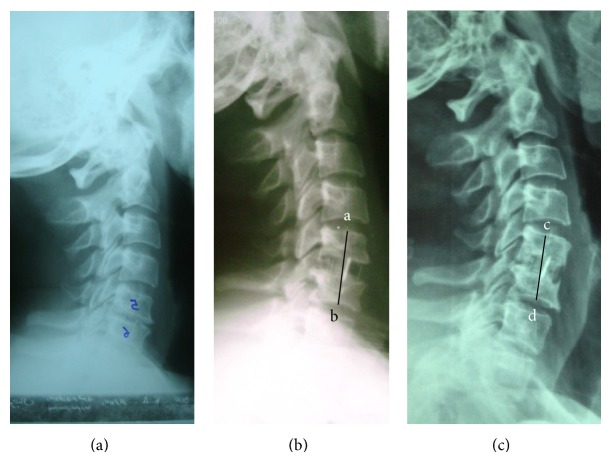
Radiographies are related to a 42-year-old female patient that presented with C5-C6 radiculopathy. ((a) Preoperative lateral view, (b) immediate postoperative view, and (c) view 41 months later.) When magnification is taken into account, 4 mm of cage subsidence (ab-cd) was found.
